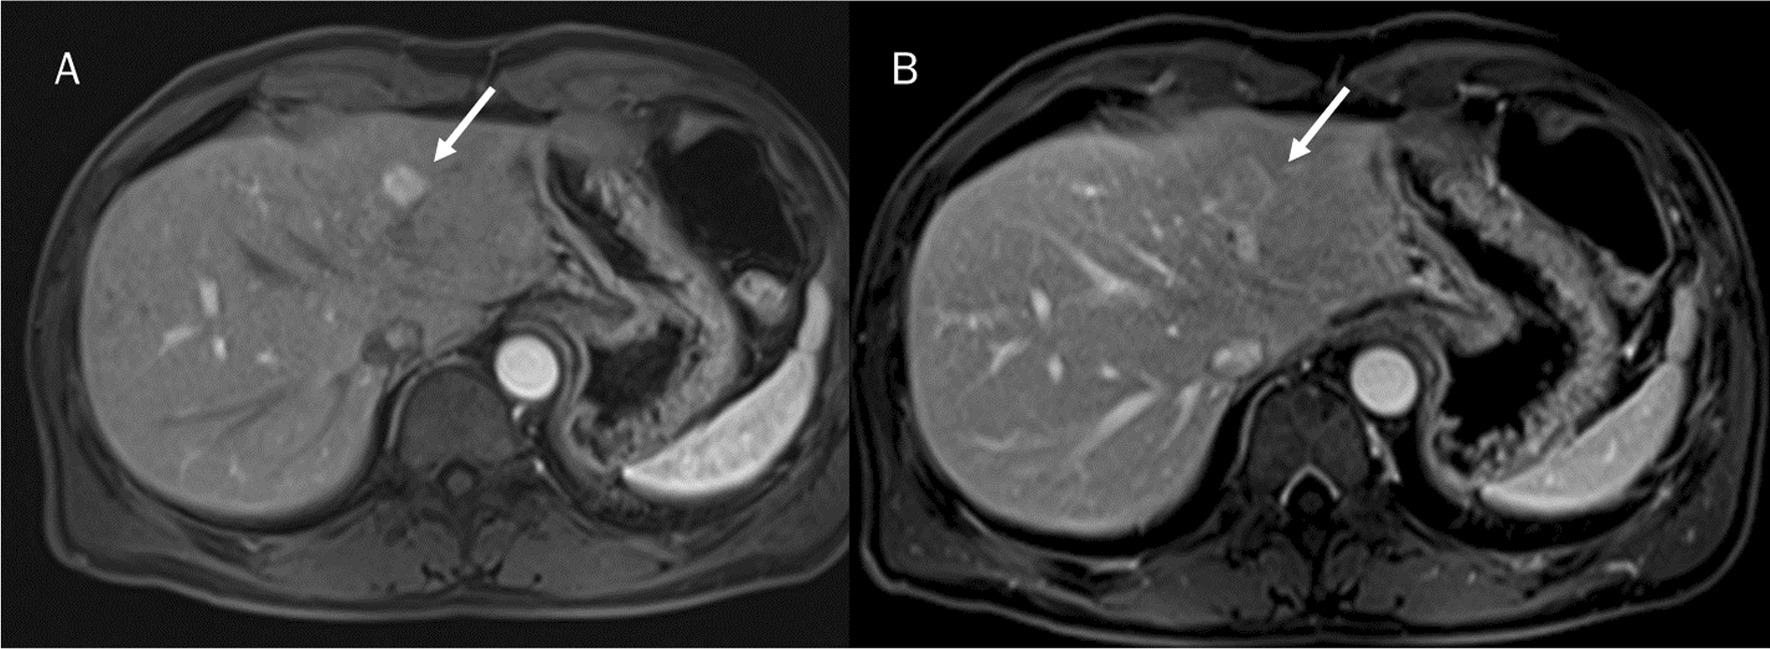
HCC on II liver segment assessed by MRI. In A, arterial phase, the arrow shows lesion arterial hyperenhancement. In B, portal phase of contrast study, the arrow shows lesion wash-out and capsule appearance
